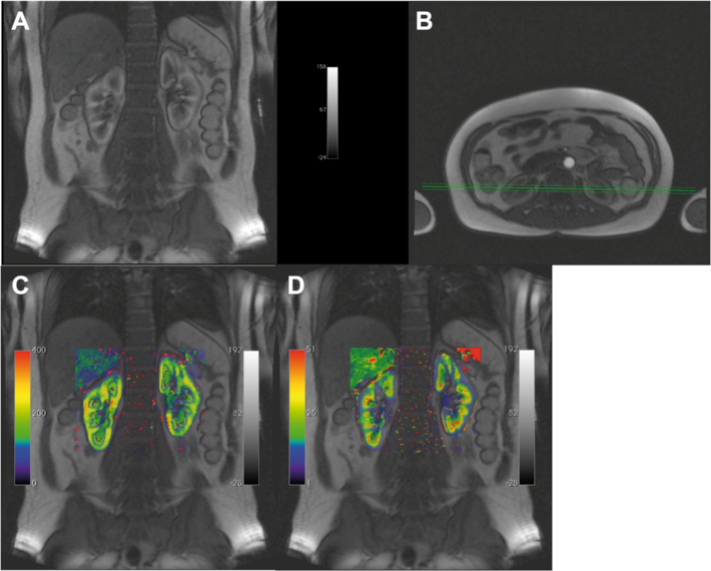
Example using a data set with different slice orientation and UMMperfusion. a and b two slices from the DCE-MRI acquired following the protocol described in [57], comprising four coronal slices (a) and one transversal slice (b). c map of the plasma flow calculated by UMMPerfusion and the 2CFM superimposed on (a, d) map of the extraction fraction derived superimposed on (a), respectively
